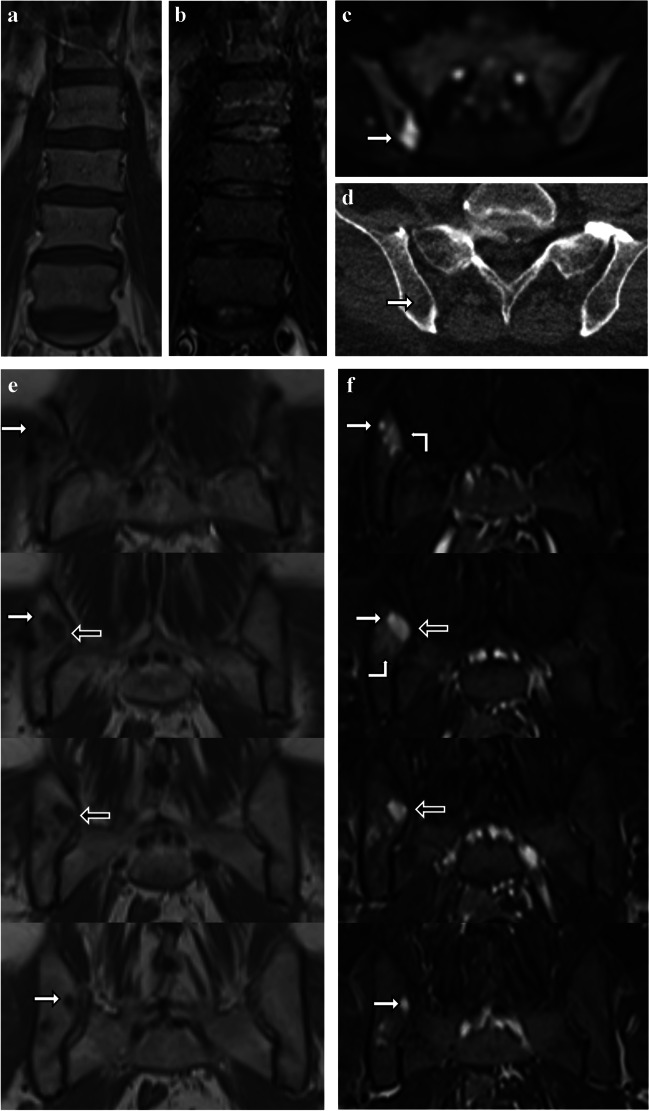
a–f Case 2. A 62-year-old male with multiple myeloma after coincidental biopsy of a small focal lesion. a, b Coronal T1tse (a) and STIR (b) MR images show physiologic signal of the bone marrow in the spine and pelvis. c, d The focal lesion shows an increased signal in b800 diffusion weighed imaging (c) and an ill-defined, trabecular rarefication in CT imaging (d). e, f Four consecutive coronal T1tse (e) and STIR (f) MR images from dorsal (upper MR images) to ventral show a small circular area (white arrows) with marked T1-hypointense and marked STIR-hyperintense signal corresponding to the biopsy channel. The biopsy channel (black arrows) is passing through a spherical T1-hypointense and STIR-hyperintense focal lesion of 1.2 cm by 0.8 cm. A diffuse, ill-defined, moderate STIR-hyperintensity, which is most likely corresponding to a bone marrow edema caused by biopsy and aspiration, is located next to the focal lesion and the biopsy channel (f, right angle arrows)
